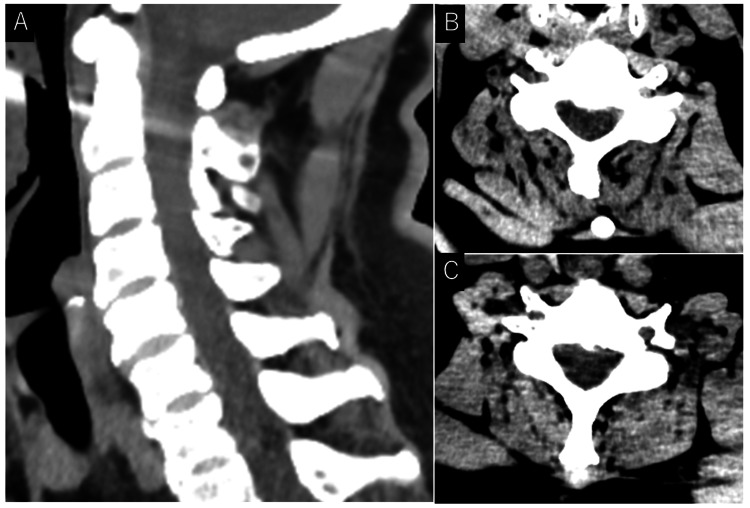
Cervical computed tomography. (A) Sagittal view and (B: C5 level and C: C6 level) axial views of cervical computed tomography showing no hematoma within the spinal canal.
